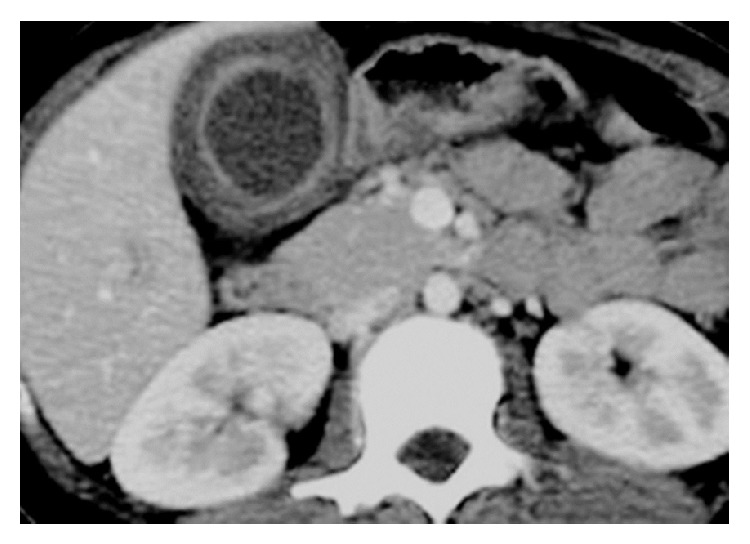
Contrast-enhanced CT showing smooth circumferential gallbladder wall thickening with a continuous contrast—enhanced mucosal line.
